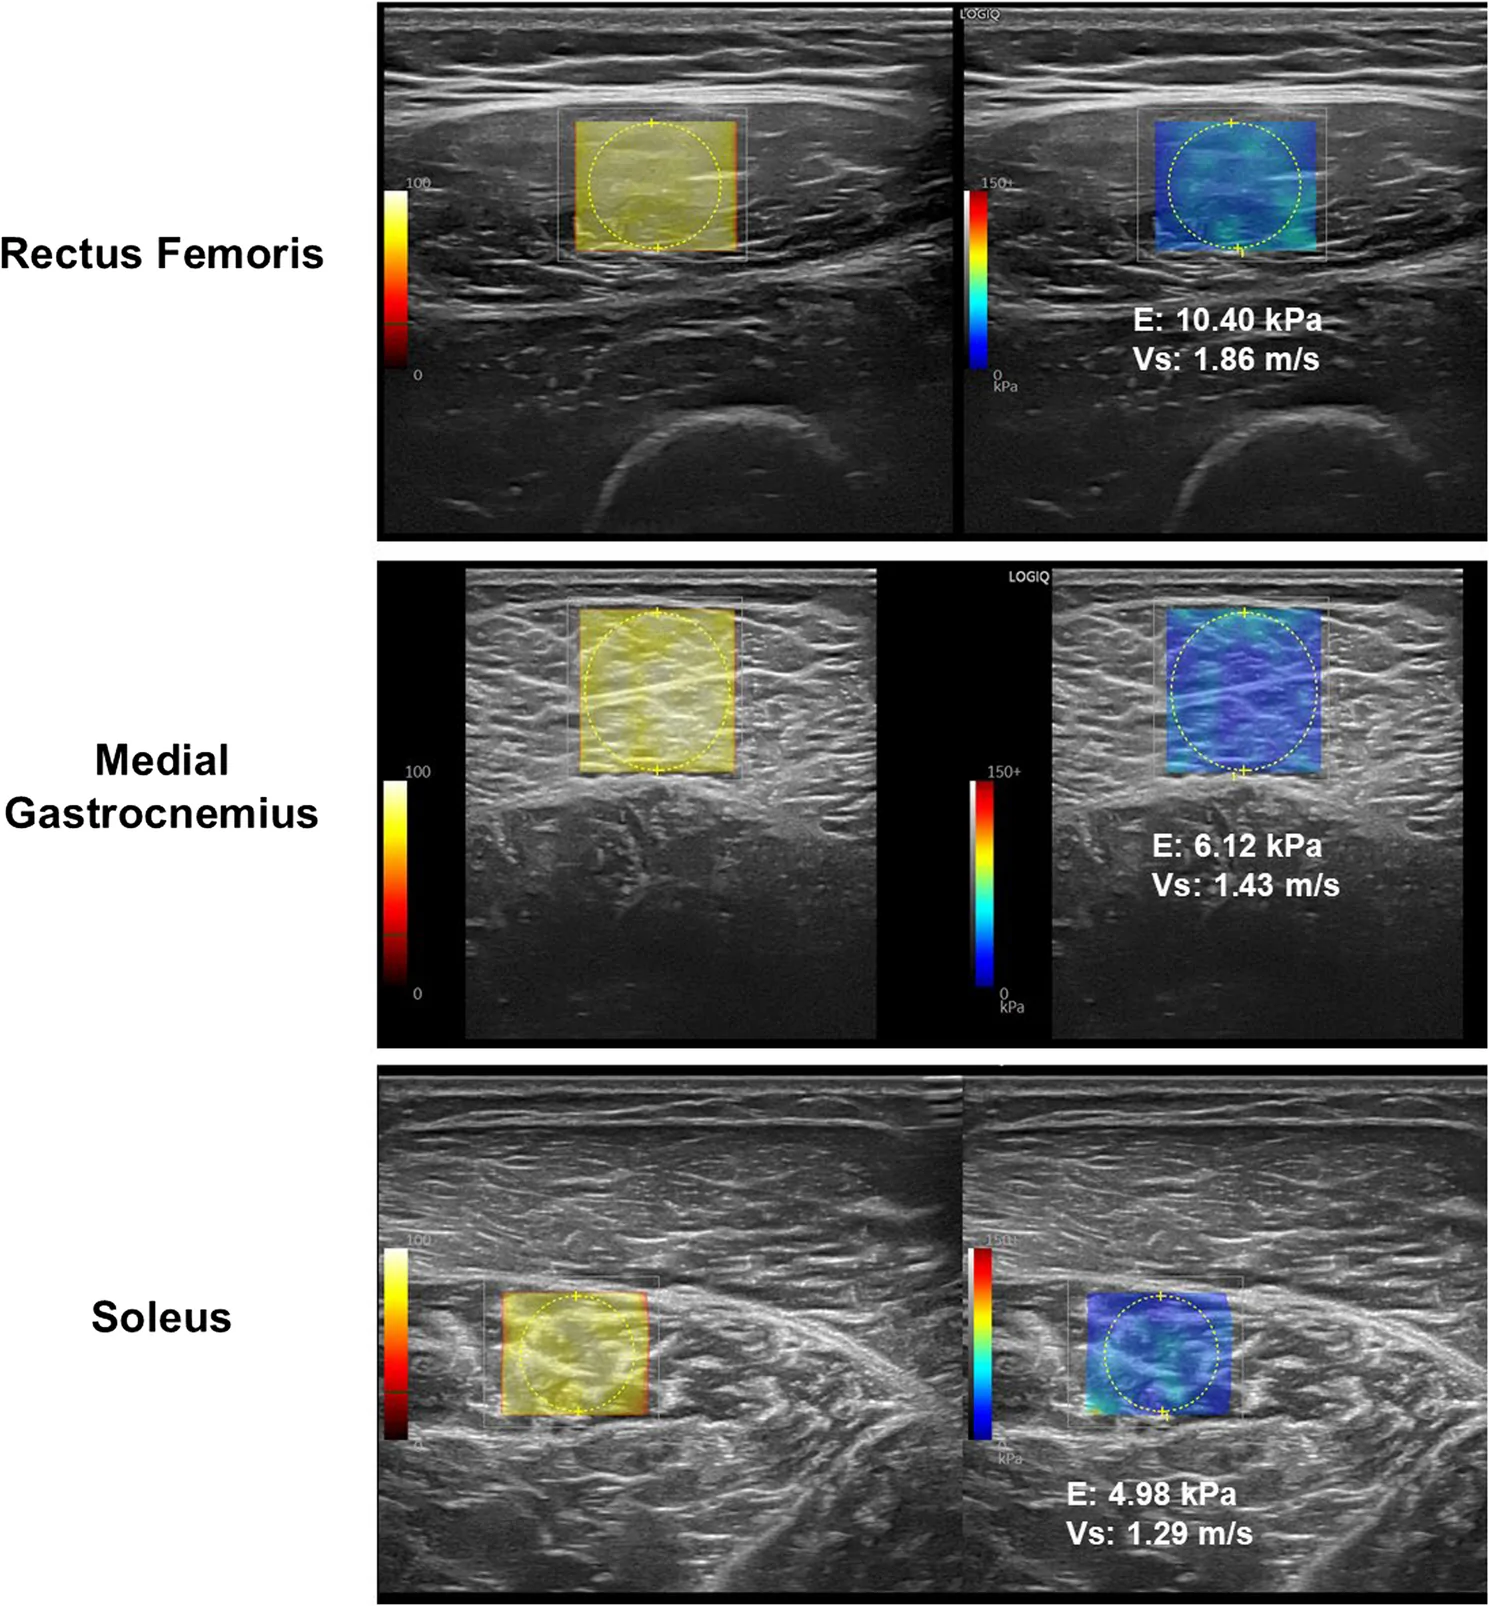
Representative SWE images of the RF, MG, and soleus muscles. Each panel displays the dual-screen format: B-mode reference image (left) with the region of interest (ROI, dashed circle) and the corresponding colour-coded elastography map (right; 0–150 + kPa scale). Mean Young’s modulus (E, kPa) and shear wave velocity (Vs, m/s) are displayed in the lower right of each panel. These values were generated automatically by the ultrasound system and have been re-typed for legibility; the numerical values are unaltered. E, Young’s modulus; Vs, shear wave velocity; kPa, kilopascal
